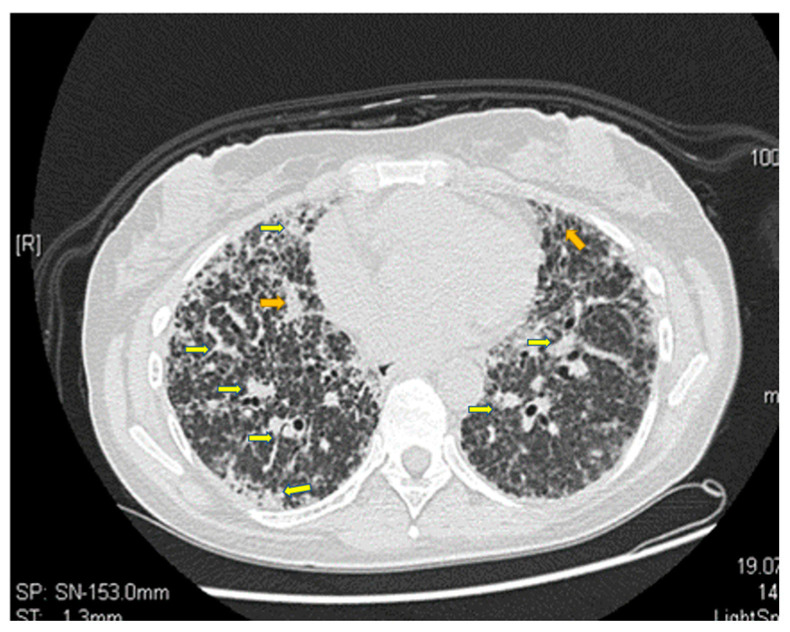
Infrahilar axial section. Polymorphous bilateral pulmonary appearance with marked augmentation with thickening of irregularly shaped interstitial septa and local pulmonary tractions. Yellow arrows indicate the problem areas. (Computed tomography scan of the lungs from the archive of the first author).
